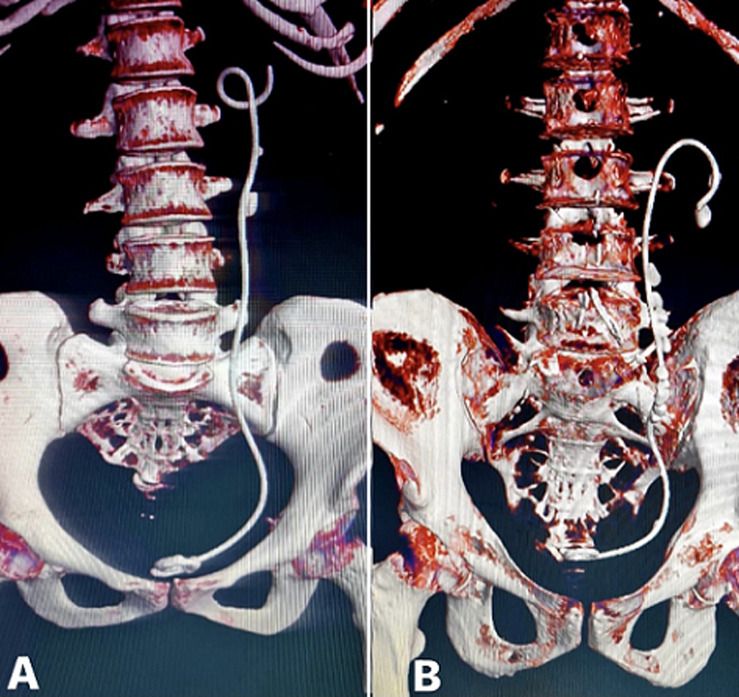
A) 3-dimensional reconstructed computed tomography images showing retained ureteral stents with encrustation of the lower coil and stone formation along the body which needed cystolithotrity and ureteroscopic lithotripsy; B) 3-dimensional reconstructed computed tomography images showing retained ureteral stents with encrustation and stone formation along the upper coil and the body which needed percutaneous nephrolithotomy and ureteroscopic lithotripsy
